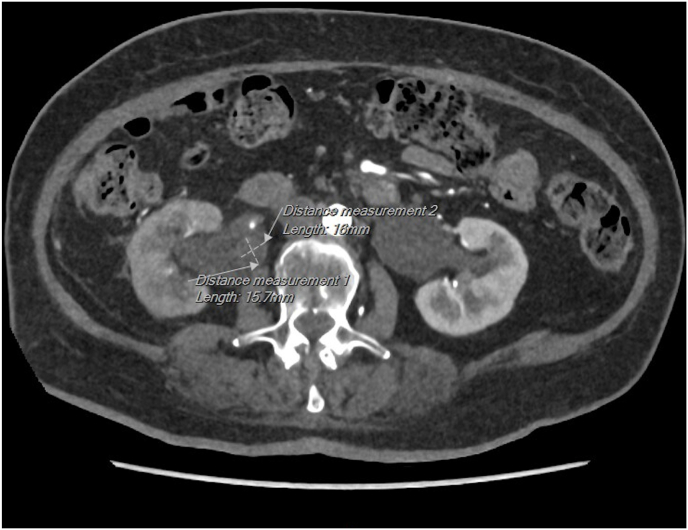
Axial (transverse) CT image in arterial phase showing a small obstructing calculus at the right ureteropelvic junction (UPJ), with associated dilation of the renal pelvis and calyces. The stone is measured in two dimensions.
